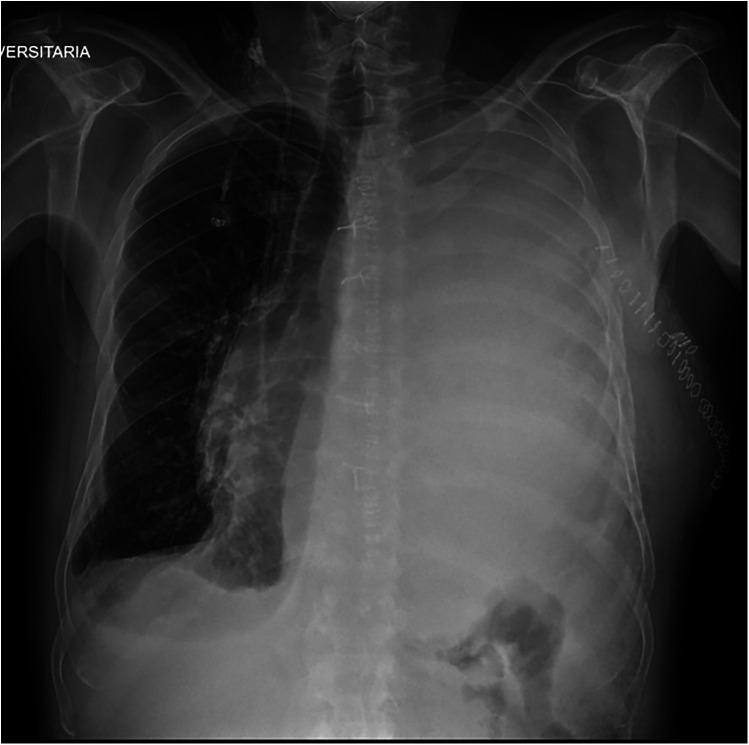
Chest x-ray showing massive left pleural effusion.
